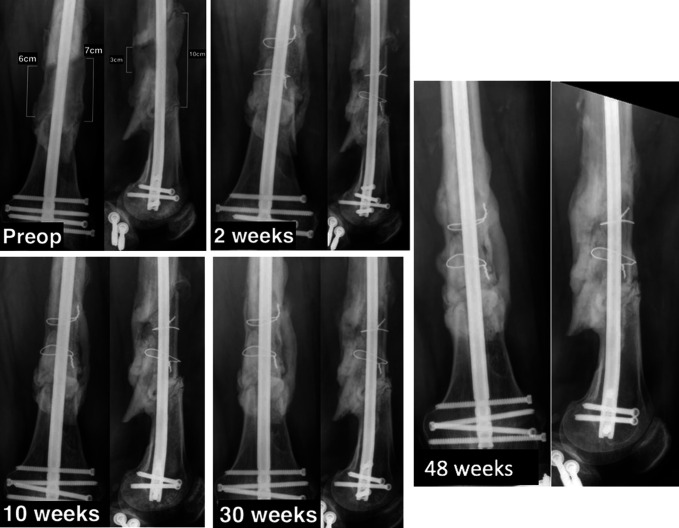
Case 1. Preoperative, 2 weeks, 10 weeks, 30 weeks, and 48 weeks follow-up radiographs.
